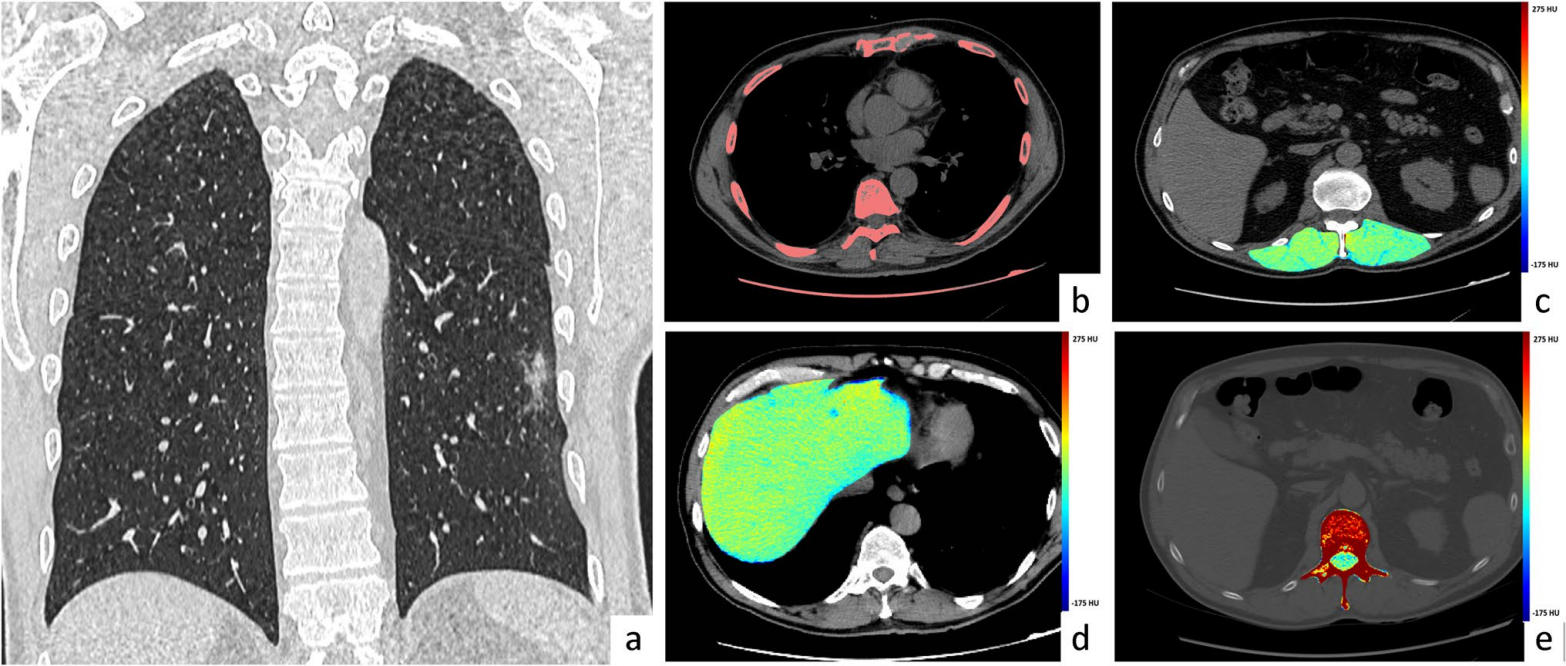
Exemplifying patients treated without the need for oxygen therapy. Male patient, 56 y.o. Vital signs were stable at the moment of admission to the hospital, and SaO2 in ambient air was 95%. C-Reactive Protein was 1.15 mg/dL. CT scan was performed two days after admission and showed a minimal lung parenchymal involvement with few patchy areas of ground glass opacity (Pneumonia score 1, a); moreover, the CT scan revealed absent coronary calcium (Agatston score, b), along with the absence of myosteatosis (c), liver steatosis (d), and osteoporosis (e), based on mean tissues attenuation enhanced by colorimetric maps of HU documenting HU in range of normality for paravertebral muscles (48 HU, c), liver (51 HU, d) and trabecular bone (181 HU, e). The patient was admitted to the hospital and discharged after three days without worsening of clinical condition and without the need for oxygen therapy
